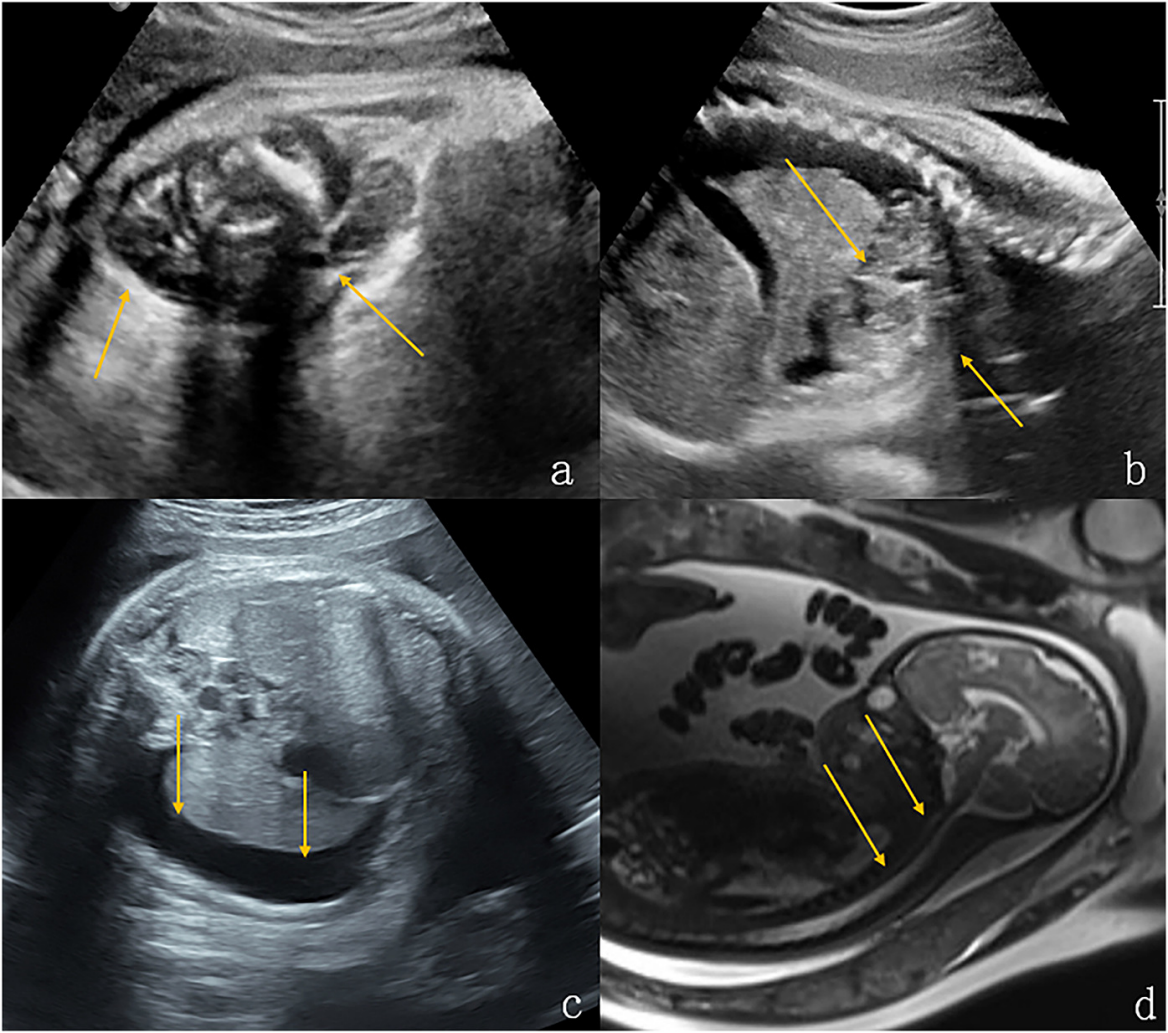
Lymphangioma in the neck and upper mediastinum of a fetus (case 11). (a,b) Solid cystic masses were found in the neck and upper mediastinum of the fetus at 37 weeks gestation (indicated by the arrows). (c) There was concomitant bilateral pleural effusion (shown by the arrows). (d) Sagittal T2-weighted magnetic resonance imaging showed that the structure of the entire trachea was visible (shown by the arrows). The newborn had dyspnea after being delivered via cesarean section at 39 weeks of gestation. After conservative treatment, the size of the tumor increased and the pleural drainage effusion increased. The infant died 45 days after birth.
